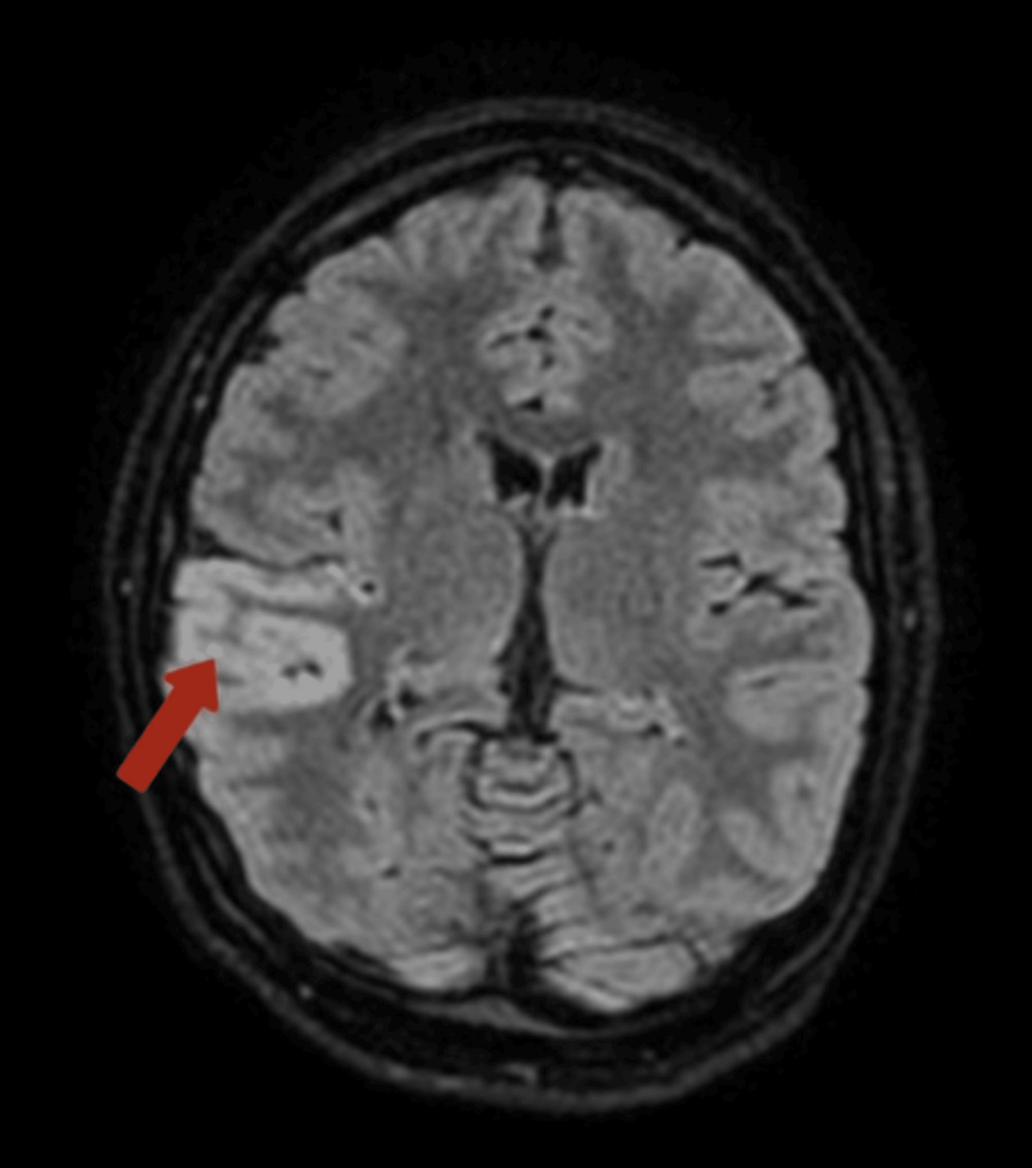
The MRI image-T2 FLAIR displays hyperintensities in the cortical and subcortical regions of the right temporo-parietal lobe. The hyperintensities observed on T2 FLAIR sequences are indicative of pathological changes, which may include edema, demyelination, gliosis, or other abnormalities. FLAIR: fluid attenuated inversion recovery
